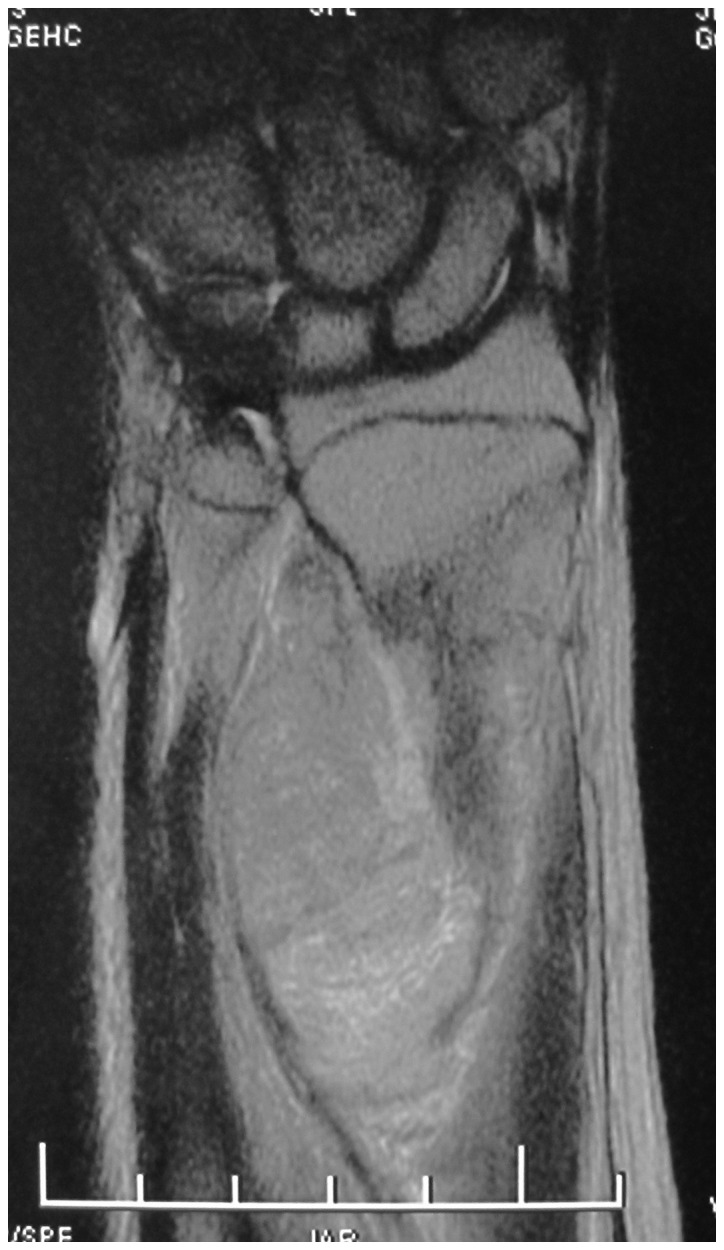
MRI revealed no epiphyseal invasion.
